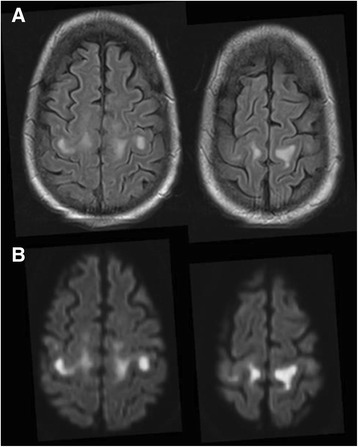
Magnetic resonance imaging in a patient with subacute flaccid tetraplegia. FLAIR images show a hyperintense signal confined to subcortical precentral gyrus on both hemispheres a. This hyperintensity is associated with high signal on diffusion-weighted imaging b, without ADC correlate (not shown)
